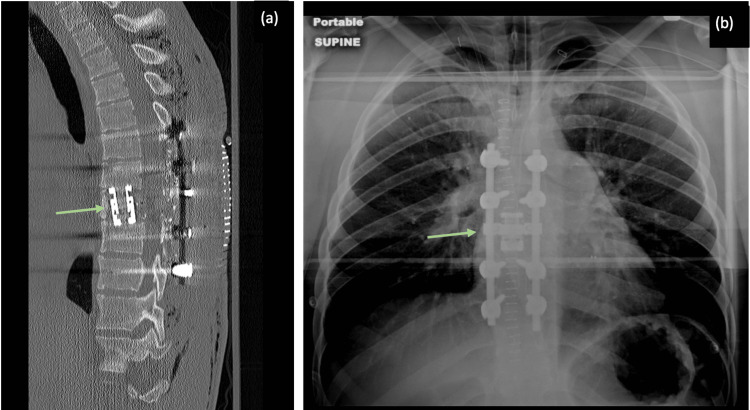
Post-operative images confirming appropriate hardware placement. (a) CT image demonstrating appropriate corpectomy cage at T8. (b) Chest X-ray demonstrating posterior hardware fixation spanning T6-T10 with corpectomy cage at T8 and laminectomies spanning T6-T9.
